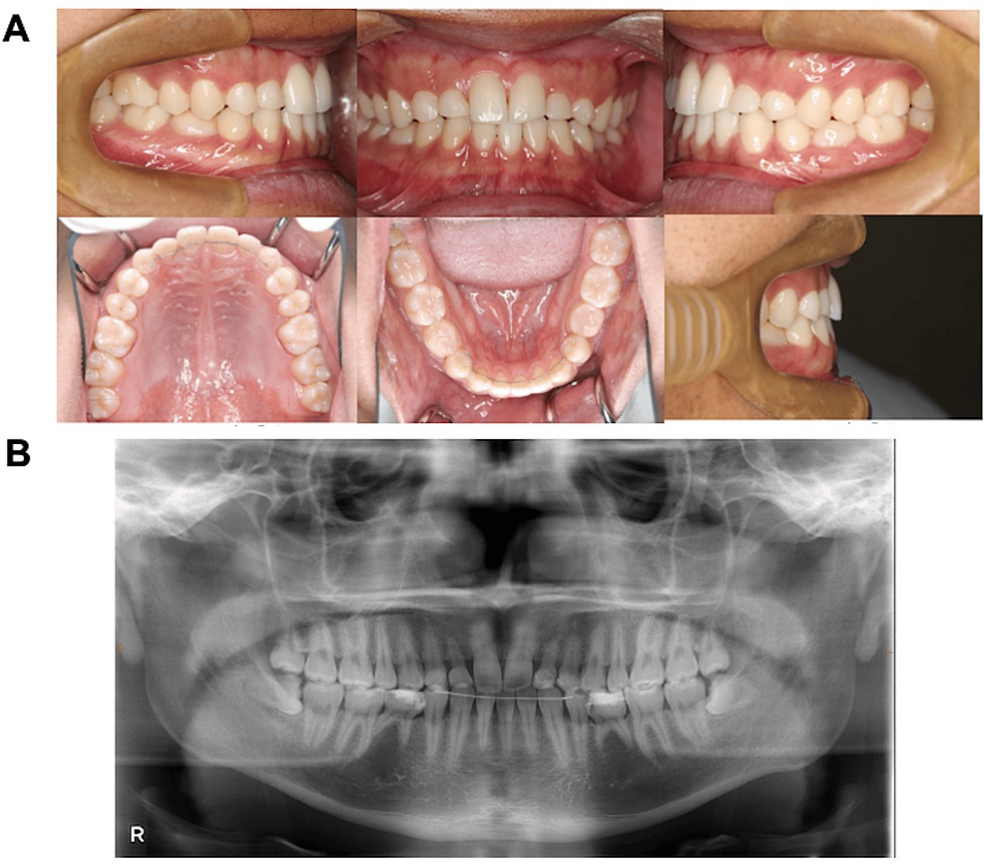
Intraoral and panoramic radiographs acquired after the conservative restorative treatment A: intraoral photographs; B: panoramic radiography
